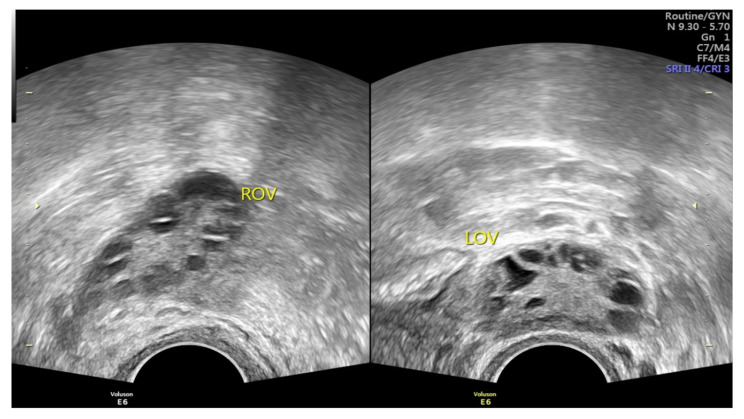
Images of PCOS under pelvic ultrasound, showing multiple immature follicles of necklace shape and/or increased volume in bilateral ovaries (Image source: Taipei Tzu-Chi hospital, Taipei, Taiwan).
